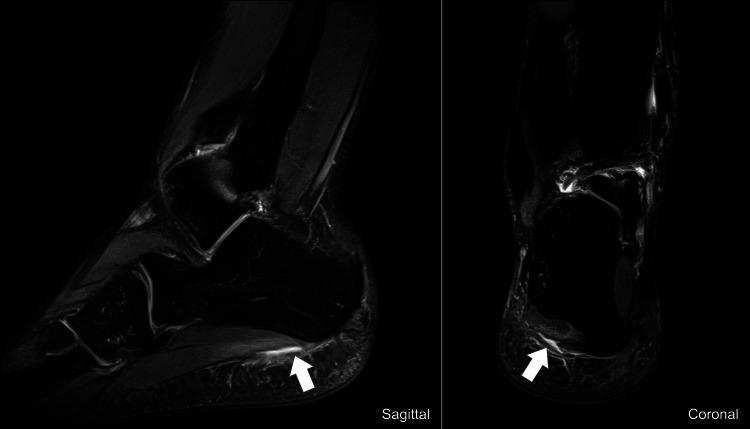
Sagittal and coronal cuts of T2-weighted MRI Note the high signal interrupting the *plantar fascia* low signal (white arrow) MRI: magnetic resonance imaging
